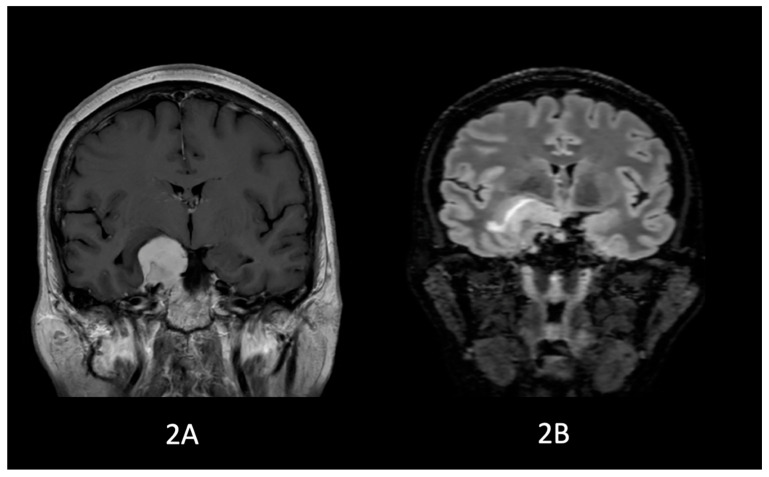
MRI study prior to surgical resection of the lesion: (1A,1B) display axial MRI images using T1-weighted sequence with gadolinium contrast and T2-weighted sequence, respectively. Note the associated edema surrounding the meningioma located in the right medial temporal lobe. Similarly, (2A,2B) present the same findings in a coronal view.
